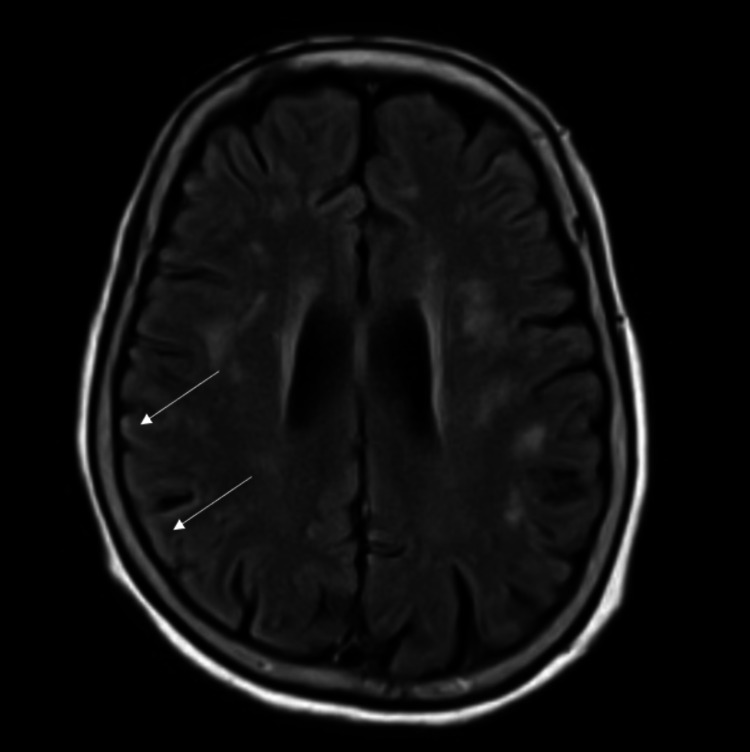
MRI brain scan showing increased FLAIR signal in the cortical sulci of the right parietal lobe (arrows). MRI = magnetic resonance imaging; FLAIR = fluid-attenuated inversion recovery
